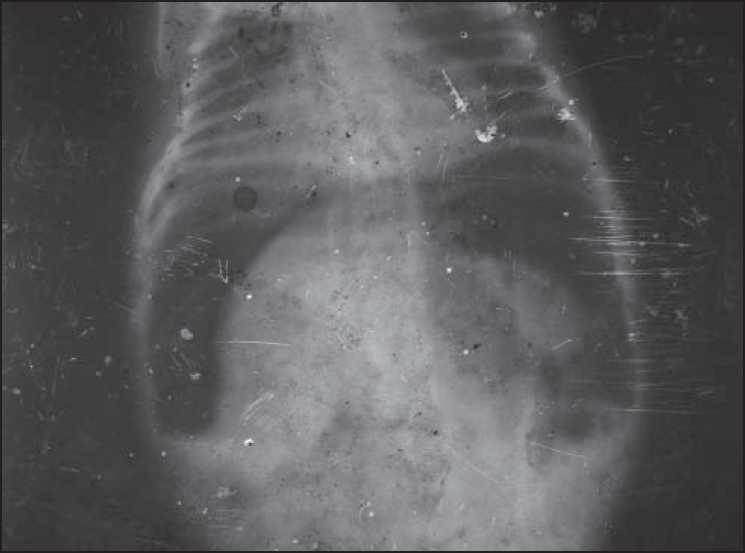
Abdominal X-ray showing large saddle-shaped shadow suggesting free peritoneal gas
